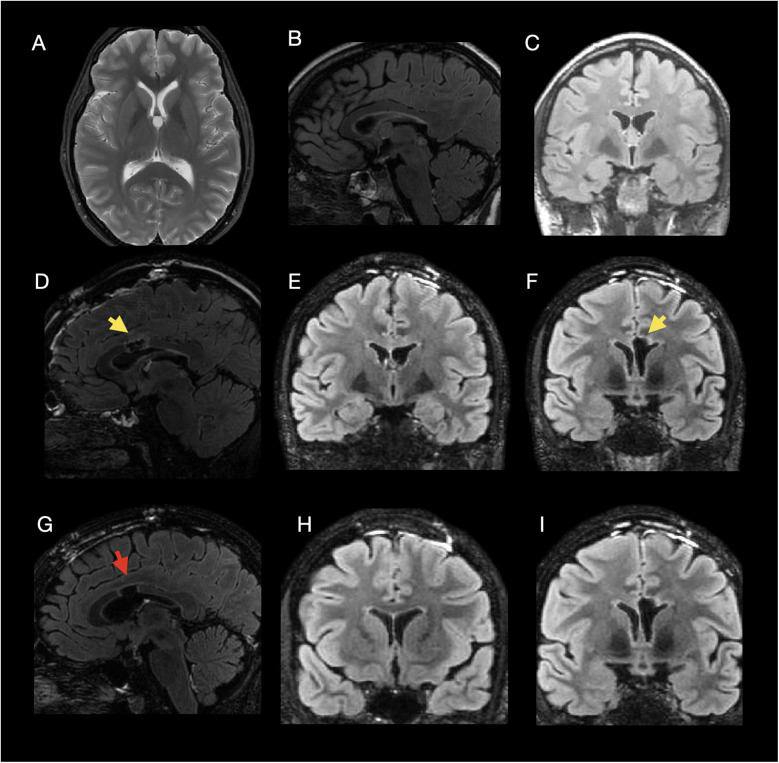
Multimodal imaging of a 27-year-old male with headache and ataxia due to a symptomatic colloid cyst, treated with endoscopic resection. Preoperative MRI (A–D): Axial (A) and sagittal (B) post-contrast T1-weighted, and sagittal (C) and coronal (D) 3D T2-weighted FLAIR images demonstrate a mildly hyperintense, well-circumscribed lesion measuring approximately 2.1 cm in maximum diameter, centered in the anterior third ventricle, consistent with a colloid cyst, causing obstructive hydrocephalus. Postoperative day 1 MRI (E–H): Sagittal (E,F) and coronal (G,H) 3D T2-weighted FLAIR images show expected postoperative changes without residual enhancement, along with moderate parenchymal hyperintensity surrounding the resection bed, consistent with postoperative edema and parenchymal injury. FouR,Month folloW,Up MRI (I–K): Sagittal (I) and coronal (J,K) 3D T2-weighted FLAIR images reveal no recurrence and demonstrate parenchymal volume loss and gliosis along the surgical tract. TwentY,OnE–Month folloW,Up MRI (L): Coronal 3D T2-weighted FLAIR image shows a new hyperintense lesion in the anterior third ventricle, consistent with interval cyst recurrence. Arrow key: Yellow arrows: postoperative hyperintensity (E–H); red arrows: parenchymal loss and gliosis (I–K); white circle: recurrent colloid cyst in the anterior third ventricle (L).
